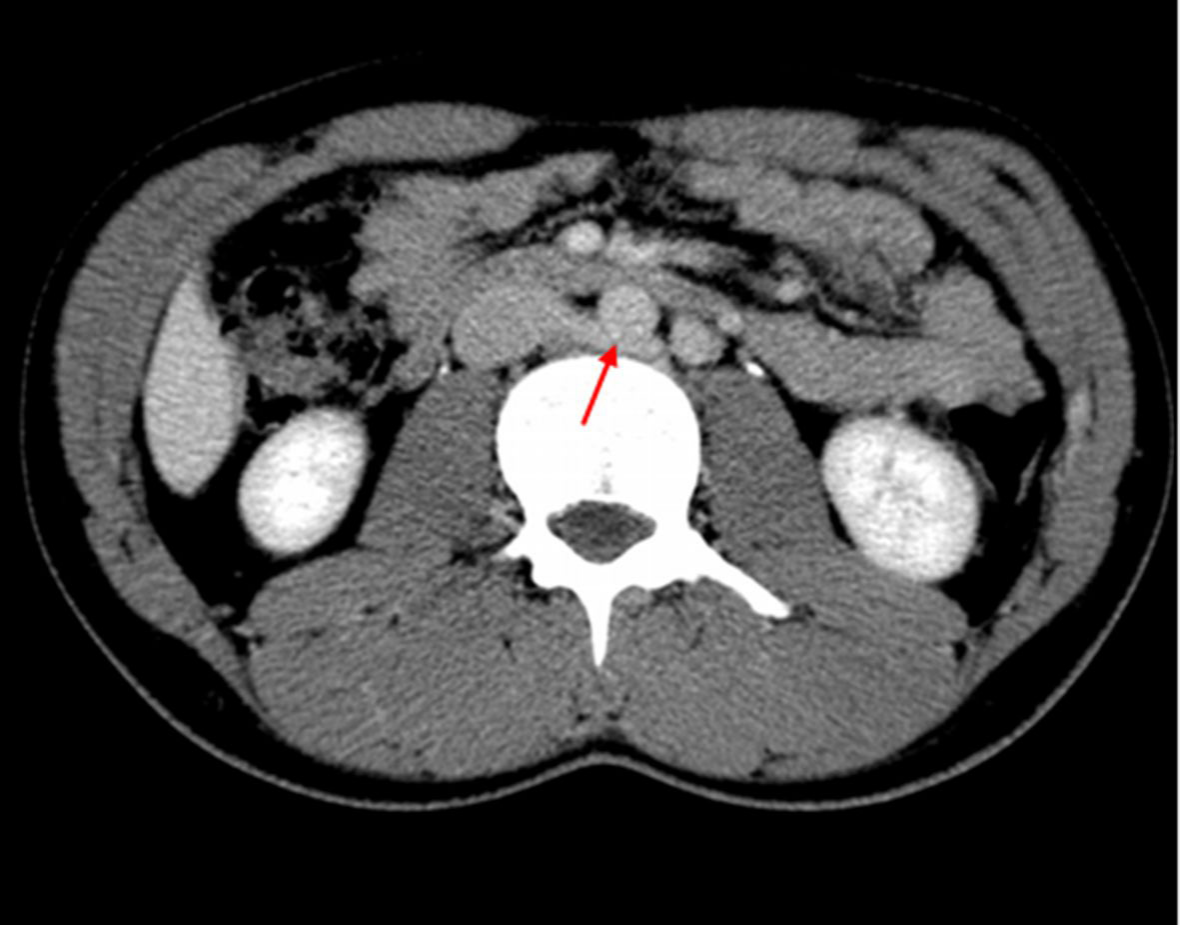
Abdominal computed tomography showing retroaortic compression of the left renal vein (arrow red). The patients’ symptomatic presentation combined with the imaging findings supported a diagnosis compatible with posterior nutcracker syndrome.
